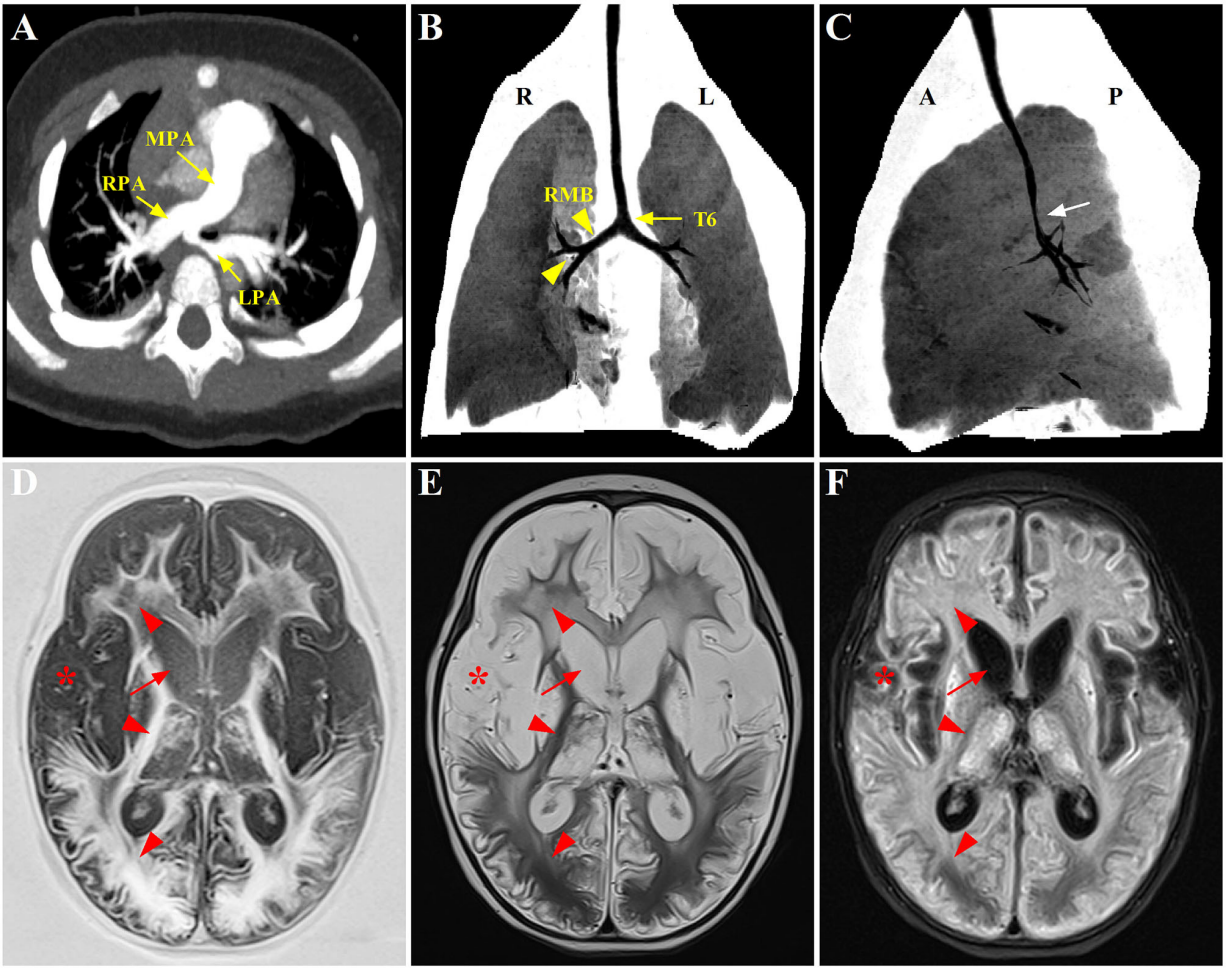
Radiographic evaluation of the PAS, tracheobronchial abnormalities, and neurological lesions through CT and MRI scans. (A) Preoperative CTA showing the anomalous origin of LPA from the posterior aspect of RPA. (B,C) Postoperative three-dimensional reconstruction of CT showing a lower level of bronchial bifurcation at T6 (yellow arrow) and a long RMB with bronchial divisions identical to left bronchial type (yellow arrowhead), associated with a stenotic segment in the distal trachea (white arrow); (D–F) Axial T1-weighted, T2-weighted, and T2-FLAIR brain MRI, respectively, displaying symmetrical signal abnormalities throughout the gray matter, white matter, and deep nuclei (red arrowhead), and extensive cortical necrosis (red asterisk), cerebrum atrophy and ventriculomegaly (red arrow). PAS, pulmonary artery sling; CTA, computed tomography angiography; LPA, left pulmonary artery; RPA, right pulmonary artery; RMB, right main bronchus; FLAIR, fluid attenuated inversion recovery.
